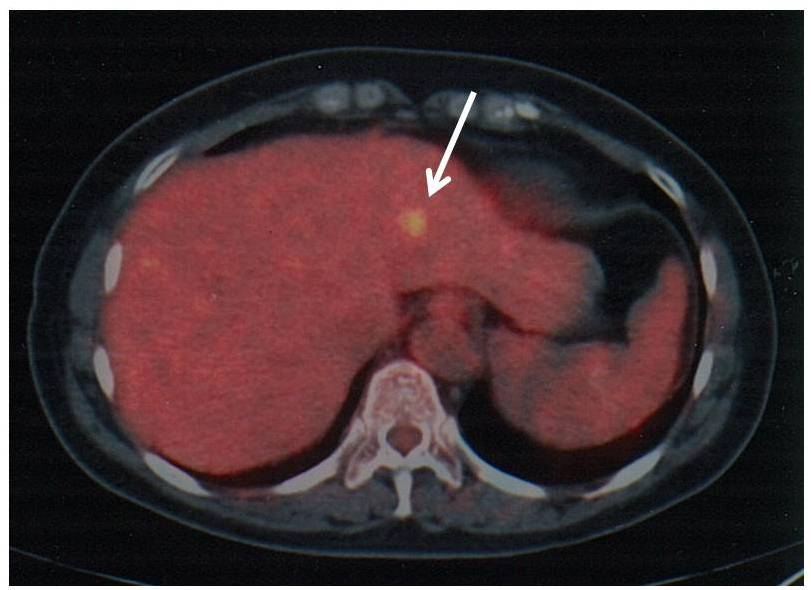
FDG-PET CT demonstrated the tumor had a high standardized uptake value (SUVmax: 3.6) for FDG. No other site showed FDG uptake, suggesting that liver tumor is not secondary from malignant lesions of other organs.
